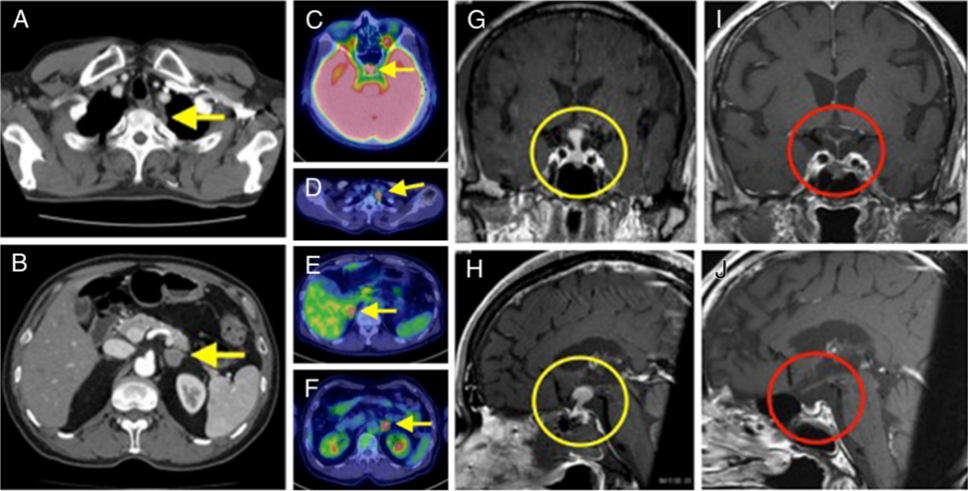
Imaging analysis. A. Chest computed tomography (CT) shows lesion at the apex of the left lung (yellow arrow). B. Abdominal CT shows a left adrenal lesion (yellow arrow). C, D, E, F. ^18^F-fluorodeoxy glucose positron emission tomography (^18^F-FDG PET) / CT. Arrows indicate high uptake in the pituitary gland (C), left lung lesion (D), and bilateral adrenal glands (E and F). G and H. Pre-treatment pituitary T1-weighted magnetic resonance imaging (MRI). G. Coronal section. H. Sagittal section. Suprasellar lesion (φ13 mm) and thickening of the pituitary stalk (circled in yellow). I and J. Post-treatment pituitary T1-weighted magnetic resonance imaging (MRI). I. Coronal section. J. Sagittal section. After chemotherapy, pituitary lesion disappeared (circled in red).
